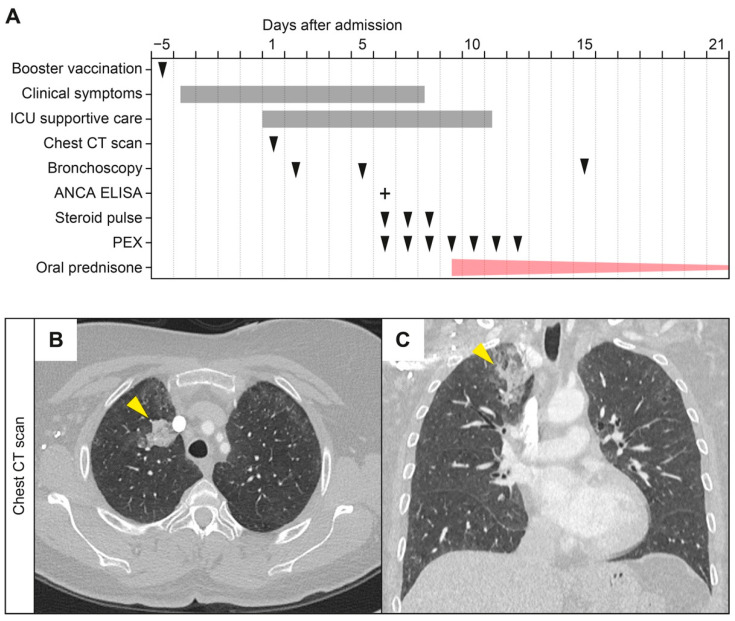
Time course of the case and radiographic findings. (A) Time course of booster vaccination, admission, performance of bronchoscopy, chest CT scan, and treatment regimen. (B,C) Computed tomography of the chest at the time of admission in axial and coronal reformation. At the time of admission, a CT scan confirmed a focal pulmonary hemorrhage in the right upper lobe with focal consolidation (arrowheads) and surrounding ground glass opacities. Furthermore, CT scans revealed subtle ground glass opacities in the anterior upper lobes. Abbreviations: ANCA = antineutrophil cytoplasmic antibody; CT = computed tomography, ELISA = enzyme-linked immunosorbent assay; ICU = intensive care unit; PEX = plasma exchange.
